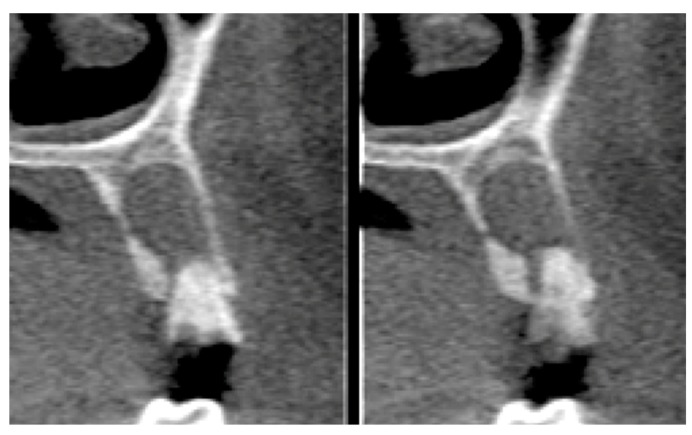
CT images of tooth 24 prior to the procedure.
